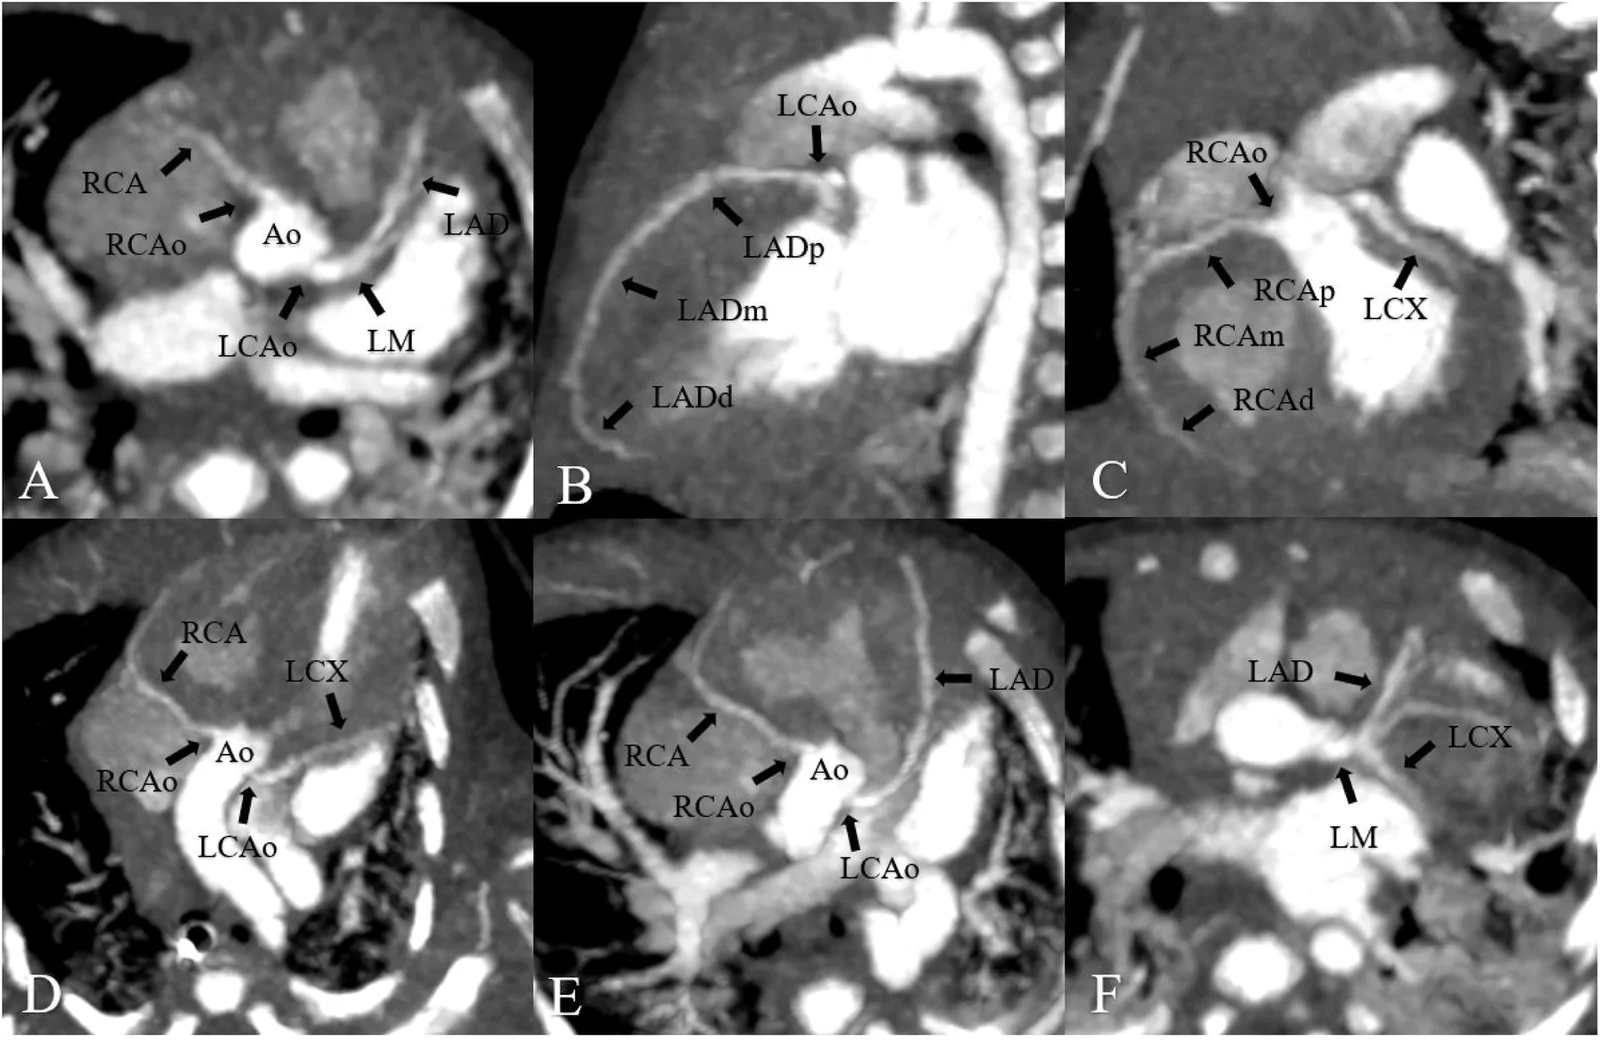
Representative coronary CT angiography images acquired using the 70-kV protocol in a neonate with ventricular septal defect. Multiplanar reconstruction (MPR), curved planar reformation (CPR), and maximum intensity projection (MIP) images demonstrate the visualization of coronary artery segments. (A) Axial image at the level of the aortic root (Ao) showing the origins of the left and right coronary arteries (LCAo and RCAo), the left main coronary artery (LM), the left anterior descending artery (LAD), and the right coronary artery (RCA). (B) Oblique sagittal CPR image demonstrating the origin of the left coronary artery (LCAo) and the proximal, middle, and distal segments of the left anterior descending artery (LADp, LADm, and LADd). (C) Oblique coronal CPR image demonstrating the origin of the right coronary artery (RCAo) and the proximal, middle, and distal segments of the right coronary artery (RCAp, RCAm, and RCAd), as well as the left circumflex artery (LCX). (D) Oblique axial CPR image demonstrating the aortic root (Ao), the origins of the left and right coronary arteries (LCAo and RCAo), the left circumflex artery (LCX), and the right coronary artery (RCA). (E) Oblique axial CPR image demonstrating the aortic root (Ao), the origins of the left and right coronary arteries (LCAo and RCAo), the left anterior descending artery (LAD), and the right coronary artery (RCA). (F) Oblique axial CPR image demonstrating the left main coronary artery (LM), left anterior descending artery (LAD), and left circumflex artery (LCX).
